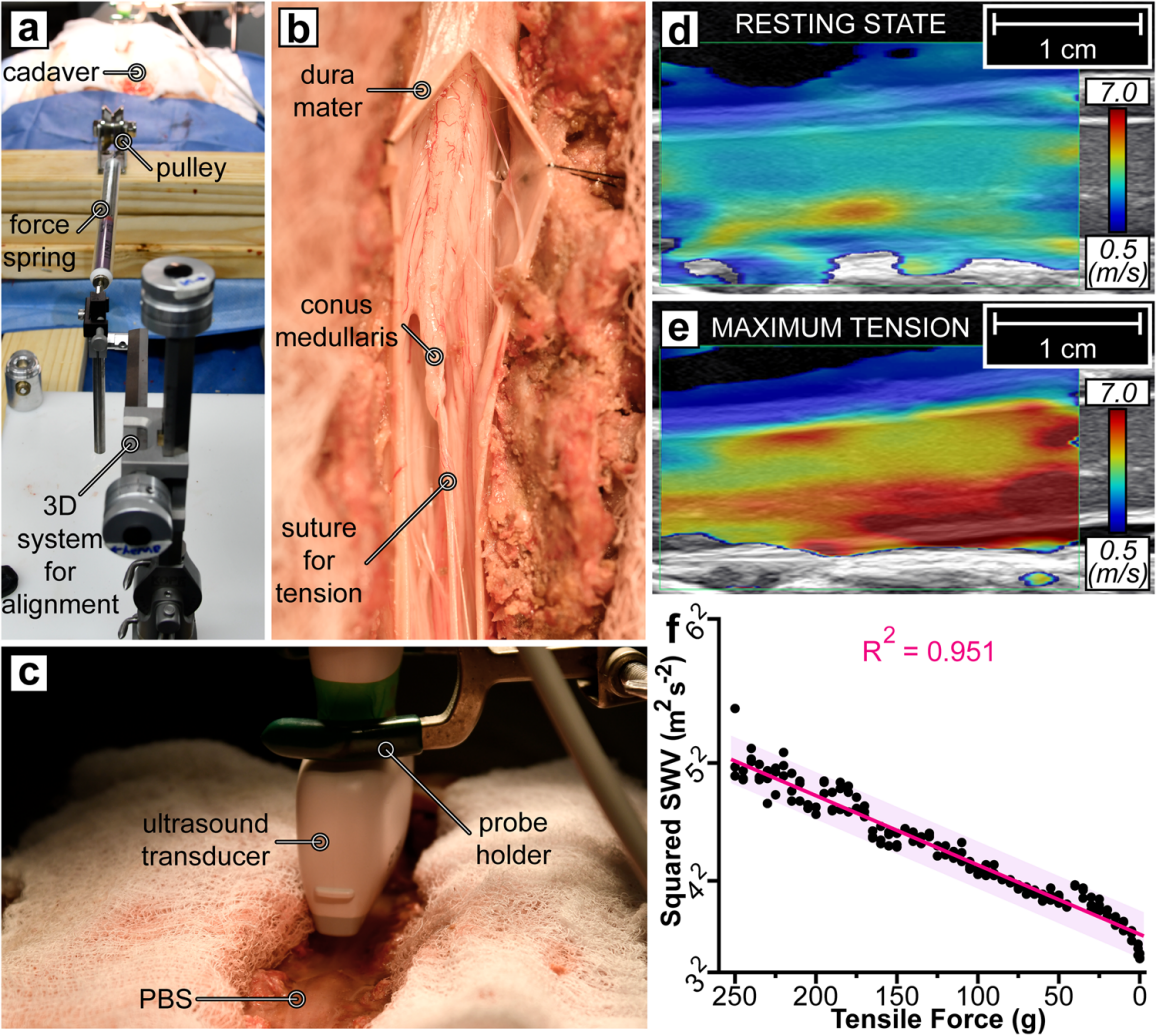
Proof-of-concept cadaveric model of ultrasound-induced shear waves as a metric for spinal cord tension. a A unidirectional tensile force was applied to the cadaveric spinal cord. The 3D stereotactic arm helped position the force gauge spring in-plane with the cord. A suture connected the spring to the spinal cord, passing over a pulley for stability and reliability. b The suture was anchored to the conus medullaris (tapered end of the spinal cord) with a Roman sandal-style knot. c The dorsal cavity was filled with phosphate-buffered saline (PBS) and the ultrasound probe was fixed in place by a probe holder. d The SWV heatmap of the spinal cord at rest and e under maximum tension of (250 g) is shown. The dura mater was not directly stretched. f From relaxation (0 and 1 g), the spinal cord was pulled at 5-increments (from 5 to 250 g), pausing for three ultrasound acquisitions at each tensile force. The squared SWV values were plotted with a linear regression best-fit line and prediction band (95%) in magenta. The values on the *x*-axis descend to visually align with the other sub-studies and clinical application of reducing tension.
